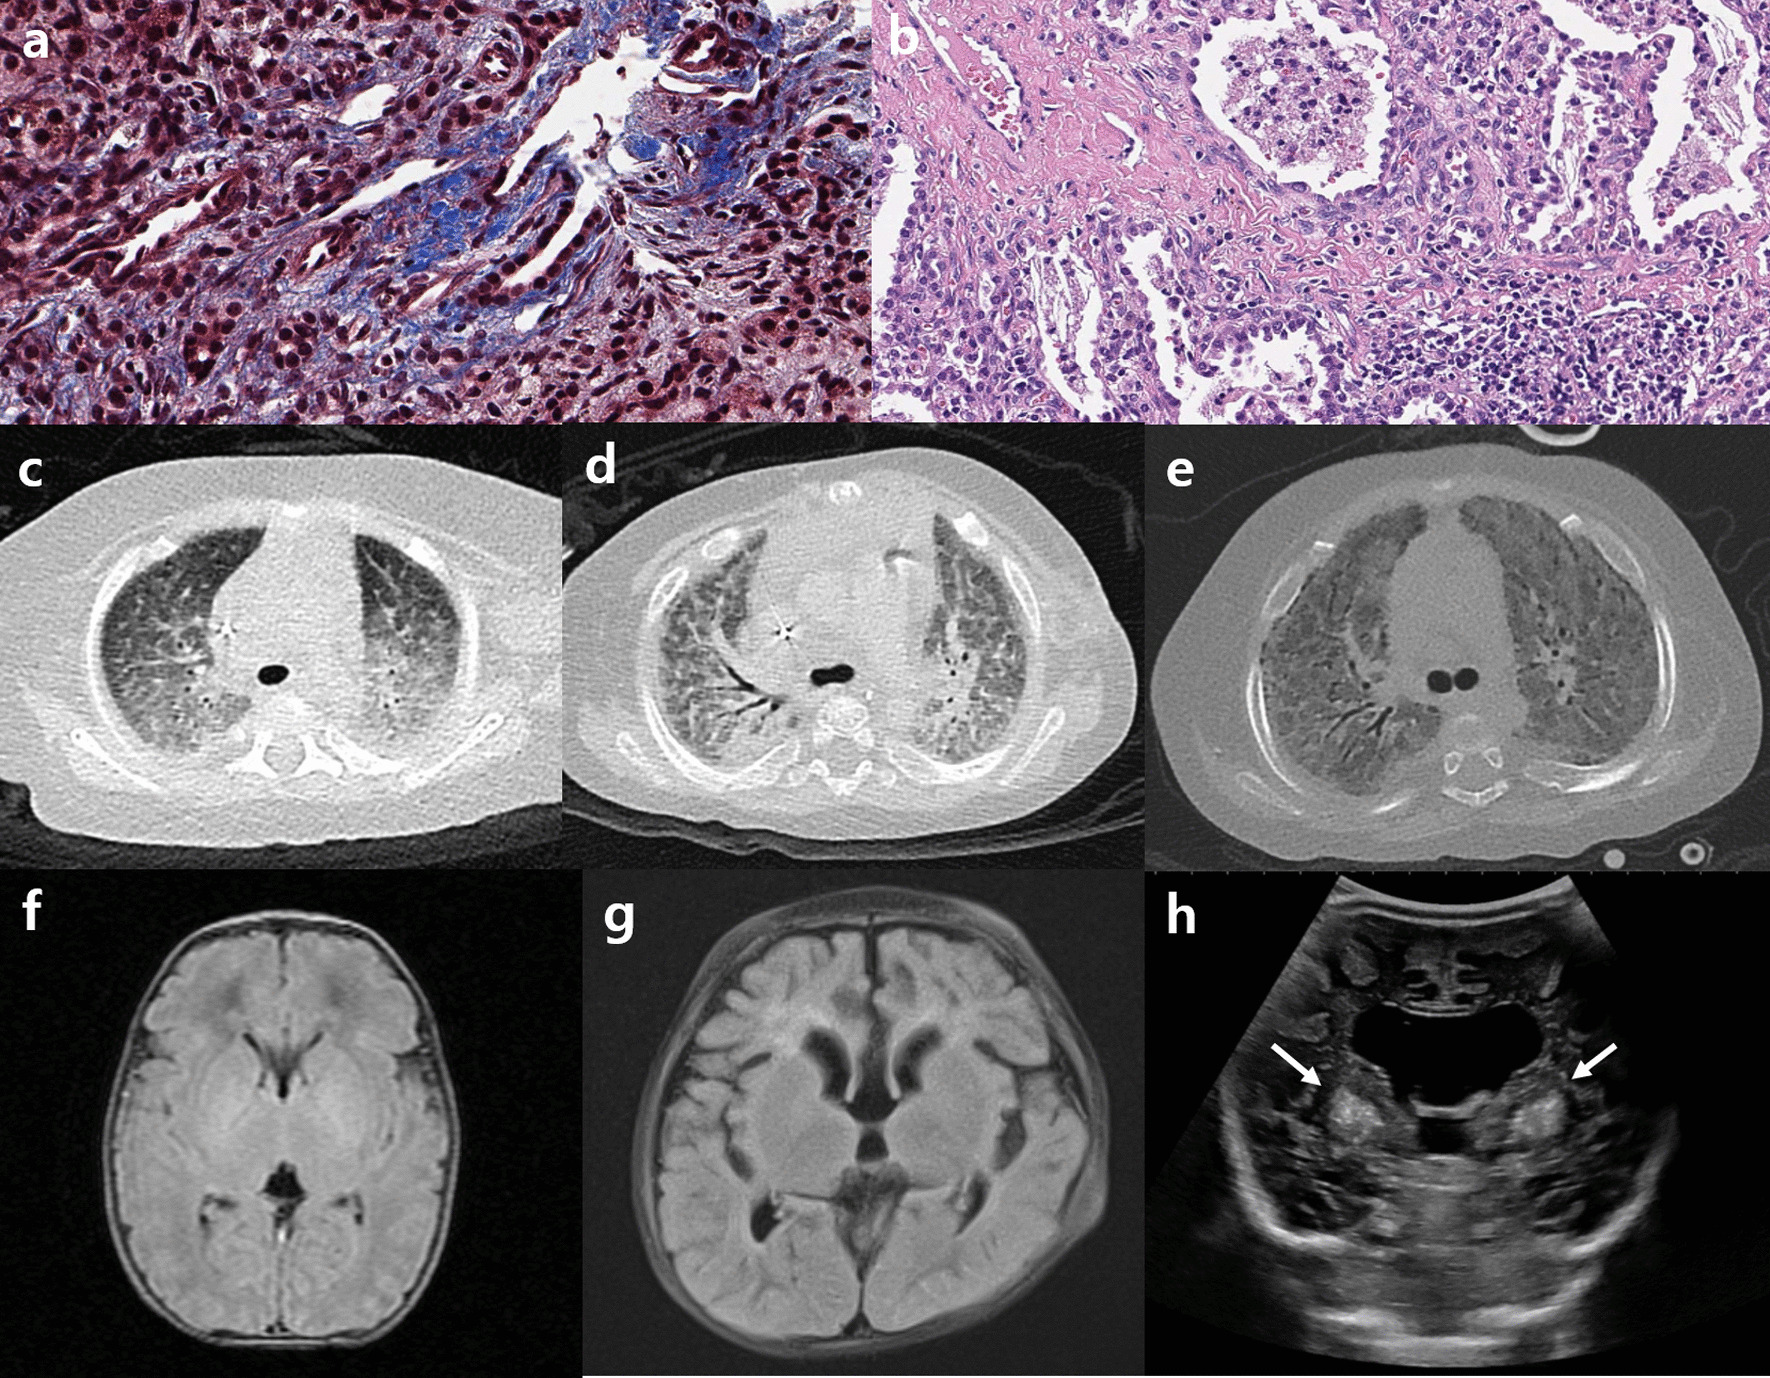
Pathologic and imaging findings of the patient. a Liver biopsy indicated periportal fibrosis and diffuse infiltration of inflammatory cells. b Lung tissues showed interstitial fibrosis with alveolar thickening. c–e Sequential chest CT revealed progressive diffuse ground-grass opacity. f–g Brain imaging indicated progressive brain atrophy, h bilateral calcifications at the basal ganglia (arrows)
